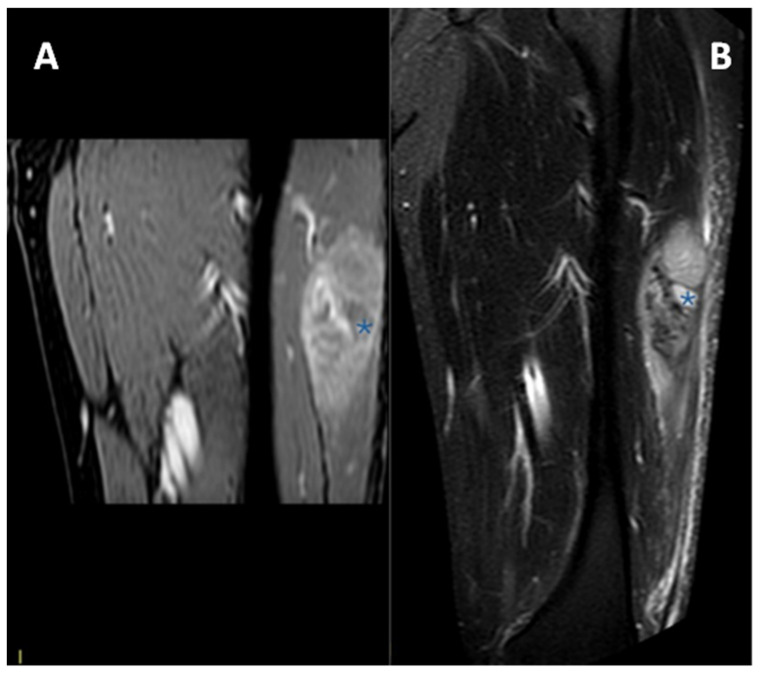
MRI in a case of deep-seated CCS of the left thigh. (A) T1 fat-saturated post-contrast coronal plane. (B) T2 fat-saturated coronal plane. A clear macroscopic area of necrosis within the lesion is appreciable (asterisks).
